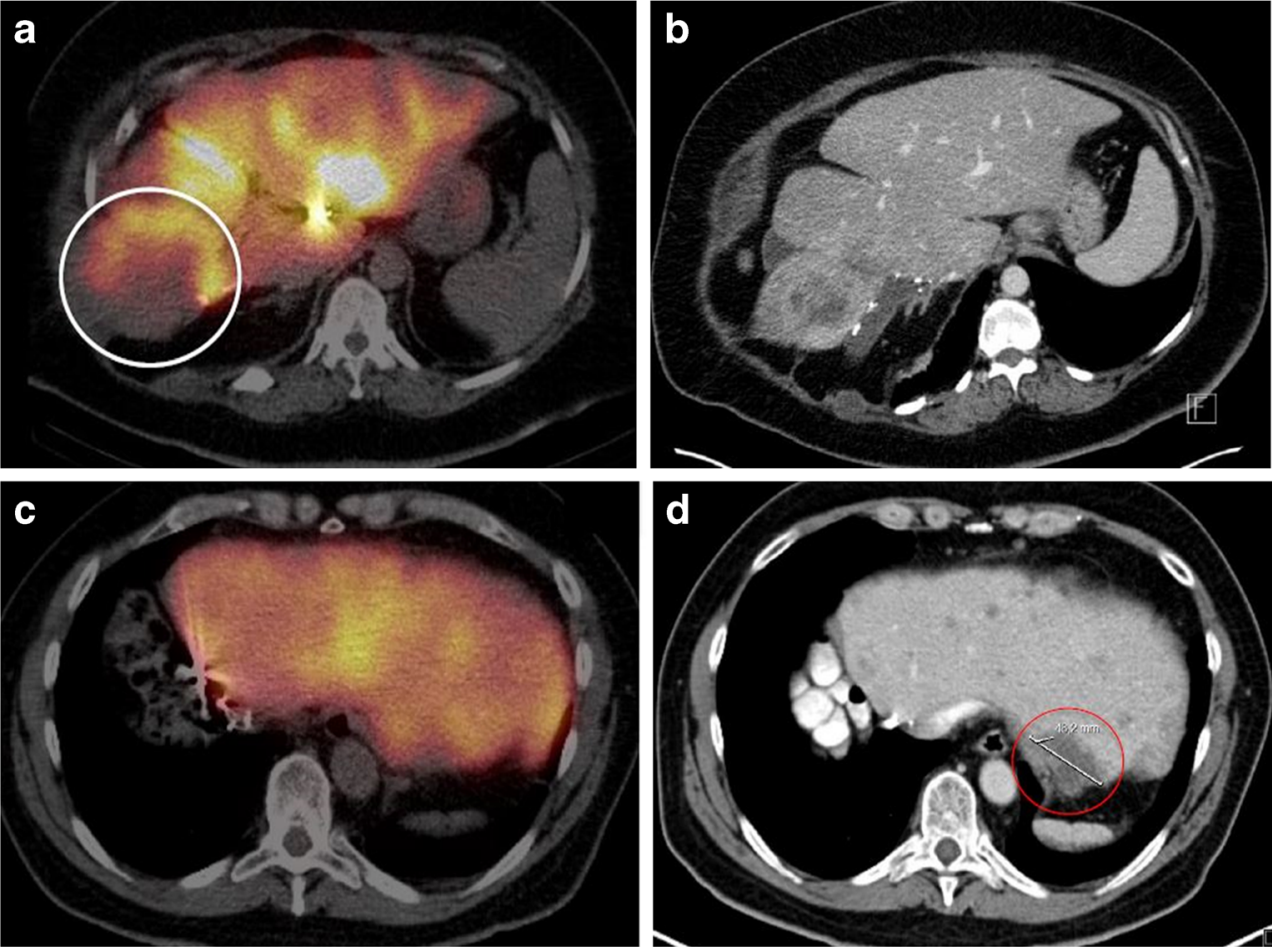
The ^99m^Tc-MAA SPECT (A and C) and the pre-procedural CT imaging (B and D) of, respectively, cases 2 and 6 as presented to the expert panel along with following information: the ^99m^Tc-MAA SPECT of case 2 shows low activity around the largest lesion in segment VIII and relatively high uptake in the healthy liver parenchyma after injection of MAA in the left hepatic artery (A and B). In case 6, the ^99m^Tc-MAA SPECT and the pre-procedural CT imaging show distribution of the activity throughout the remnant liver, although with lack of activity uptake around the largest lesion as depicted by the *red circle* (C and D).
